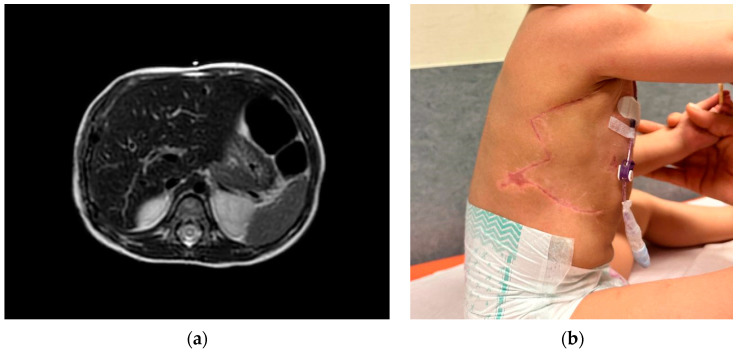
Post-operative results: (a) MRI after surgery; (b) aesthetic result.
